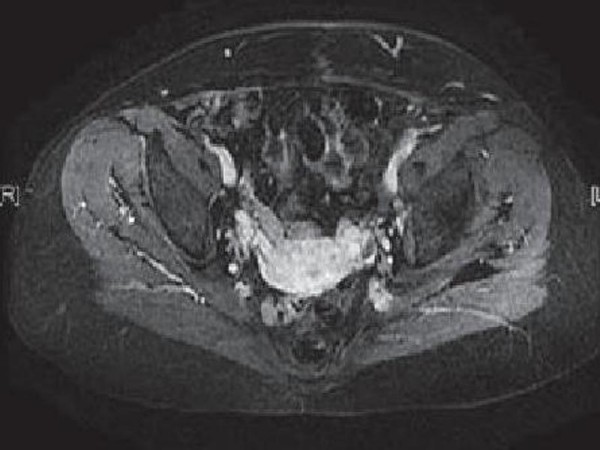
MRI showing the extent of disease after therapy: Resolution of the left pelvic mass after chemotherapy.
